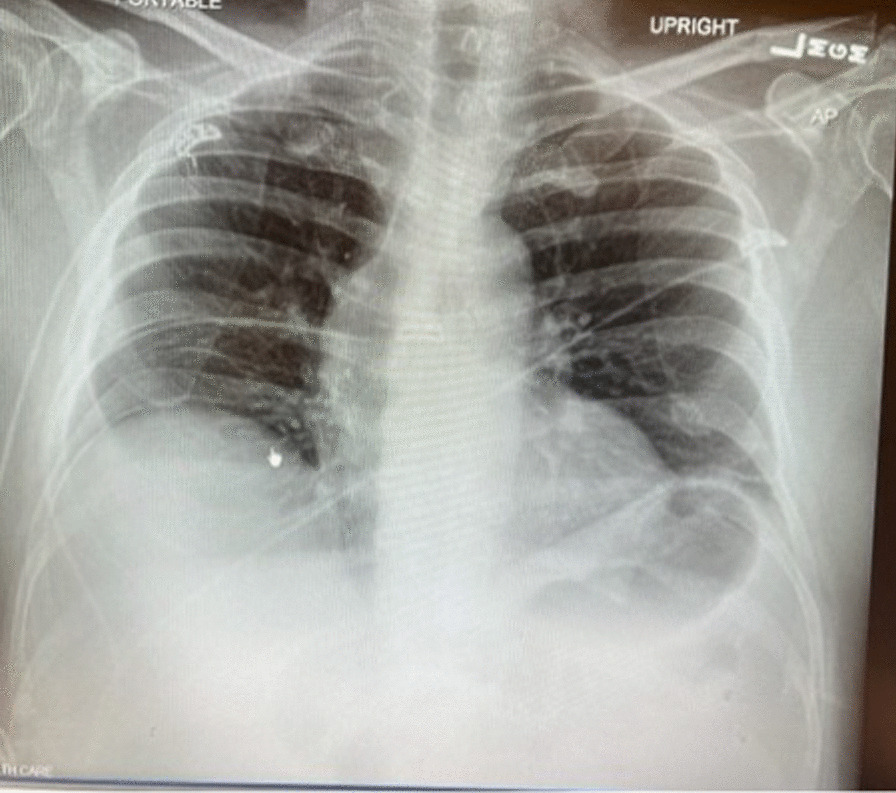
Chest X-ray upon admission showed perivascular congestion and gastric air. Cardiomegaly or significant pleural effusions were not present. Mediastinal structures appeared normal
